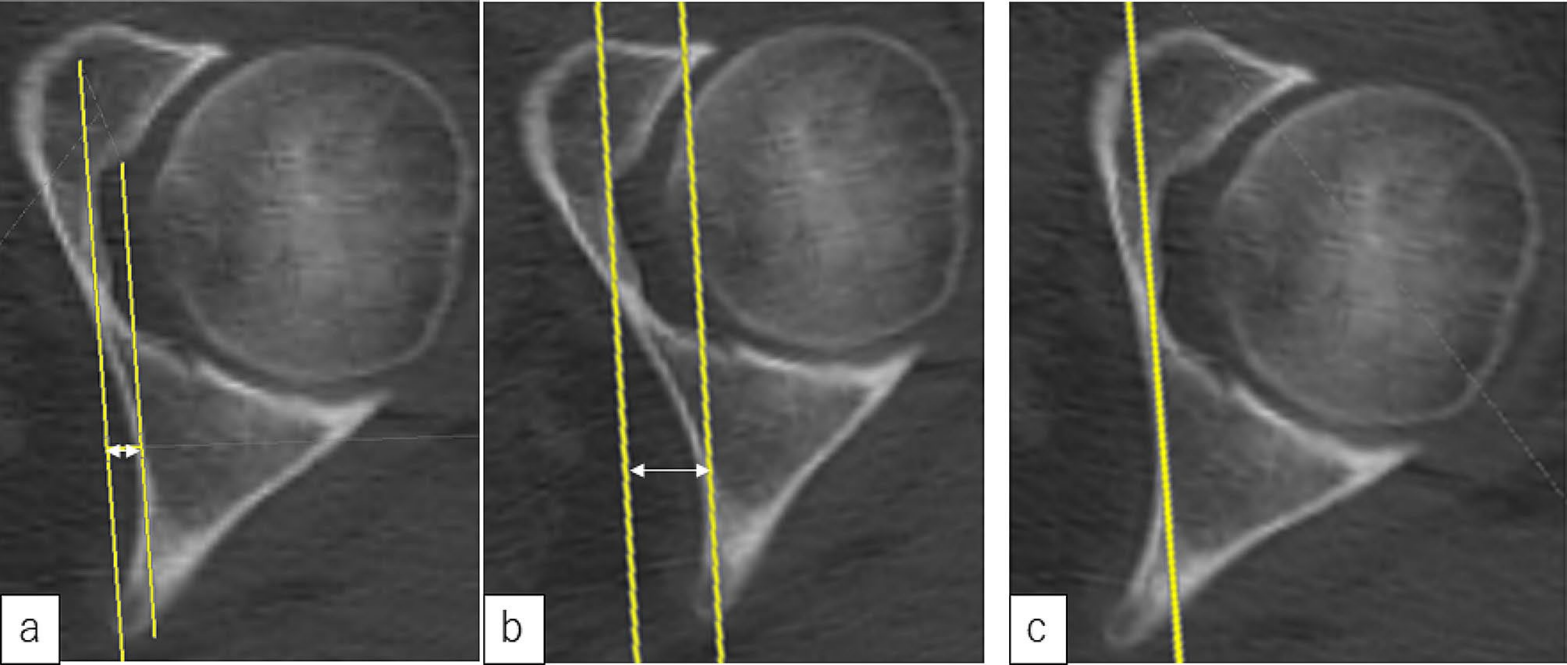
Axial CT image at the hip center level in a case of coxa profunda. a Original image. A line with a 4° incline was created to mimic the X-ray irradiation line, which is tangential to the acetabular floor and acetabular medial wall. The distance between these two parallel lines approximates the distance between the ilioischial line and acetabular floor on the anteroposterior pelvic radiograph. b The two same parallel lines were created on the original image with 10° internal rotation. The distance between the ilioischial line and the acetabular floor is increased. c The original image was rotated 10° externally. The cortical bone in contact with the line in image b is located more posterior to the ischium than in image c. CT, computed tomography
